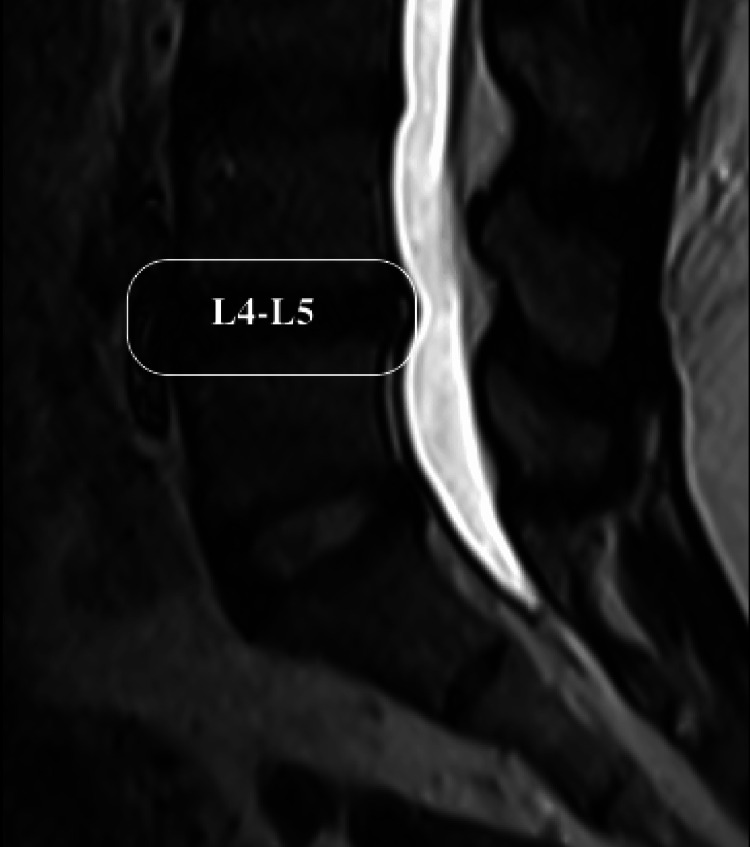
MRI sagittal T2W image of the lumbar spine demonstrating the L4-L5 intervertebral disc. MRI: Magnetic Resonance Imaging
